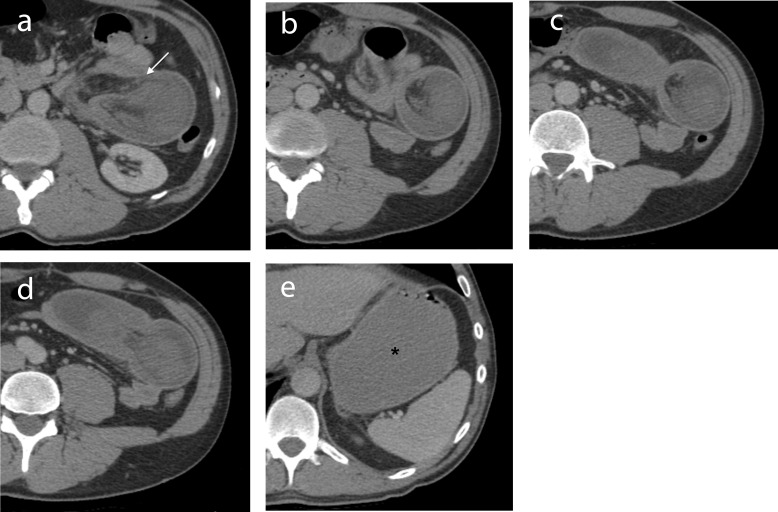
(a-d) IV contrast-enhanced axial CT images through the abdomen demonstrate a jejunal intussusception on the left side with proximal obstruction. The dilated intussuscepiens contains the intussusceptum along with mesenteric fat and vessels (arrow). (e) The stomach is distended (^*^). The 2.5 cm GIST causing the intussusception is not seen on CT.
